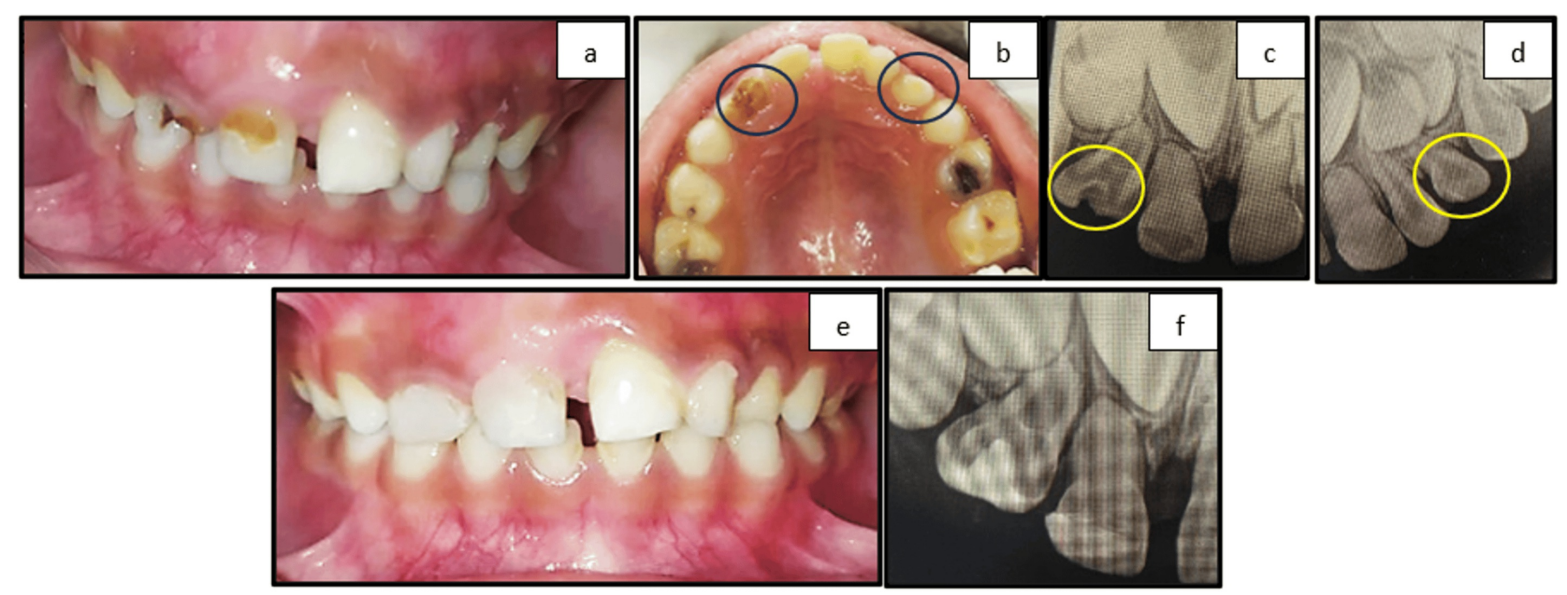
(a) Frontal view showing carious 51 and 52 and gemination of 52. (b) Maxillary occlusal view showing carious 52 and supernumerary tooth between 62 and 63. (c) Radiographic view of gemination with respect to 52. (d) Supernumerary tooth between 62 and 63. (e) Frontal view showing restored 51 and 52. (f) Radiographic view of restored geminated 52
